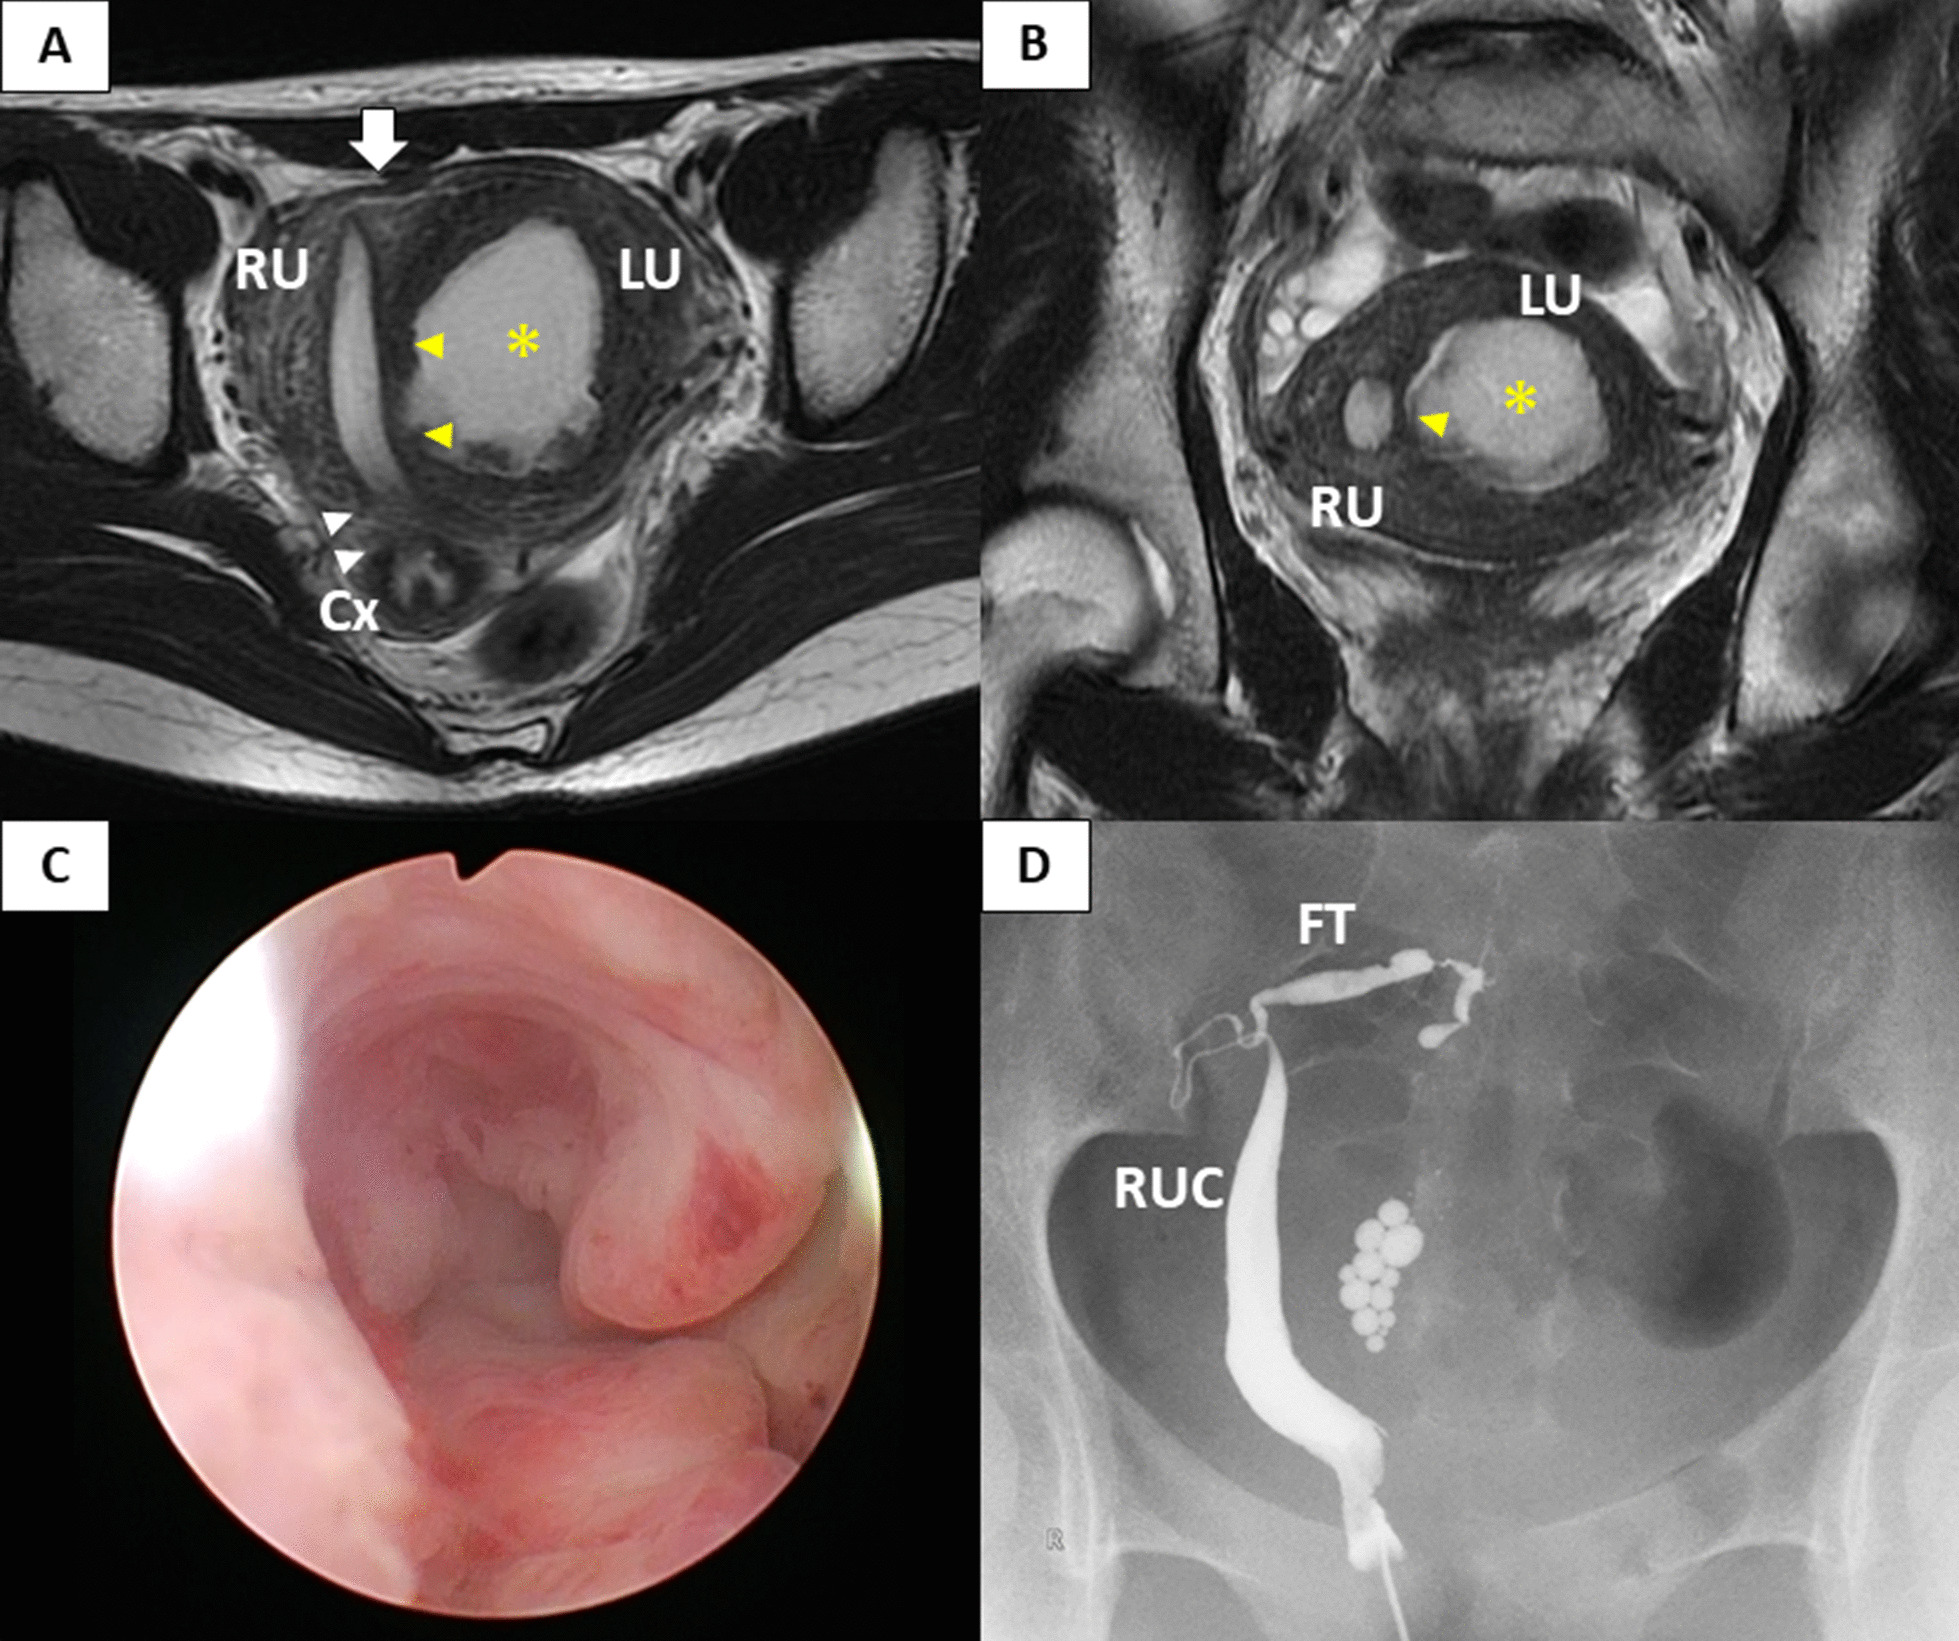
Pre-operative findings. MRI scan in axial (a) and coronal (b) planes showing the right uterus and the left uterus with a 5 cm-sized hematometra (*) in the uterine cavity. An asymmetric uterine septum is found between the left and right uterine cavity, which are not communicating (yellow triangles). One cervix is confirmed and connected to the right uterus body (white triangles). The left and right uterine fundus are clearly not divided with a normal uterine fundal contour (white arrow). Hysteroscopy in the right uterus revealed a simple small cavity, not communicating with the left uterus (c). Hysterosalpingography also showed no traffic to the left uterine cavity and the compressed shape of the right uterine hemi-cavity with fallopian tube patency (d). *RU* right uterus, *LU* left uterus, *RUC* right uterine cavity, *FT* fallopian tube
